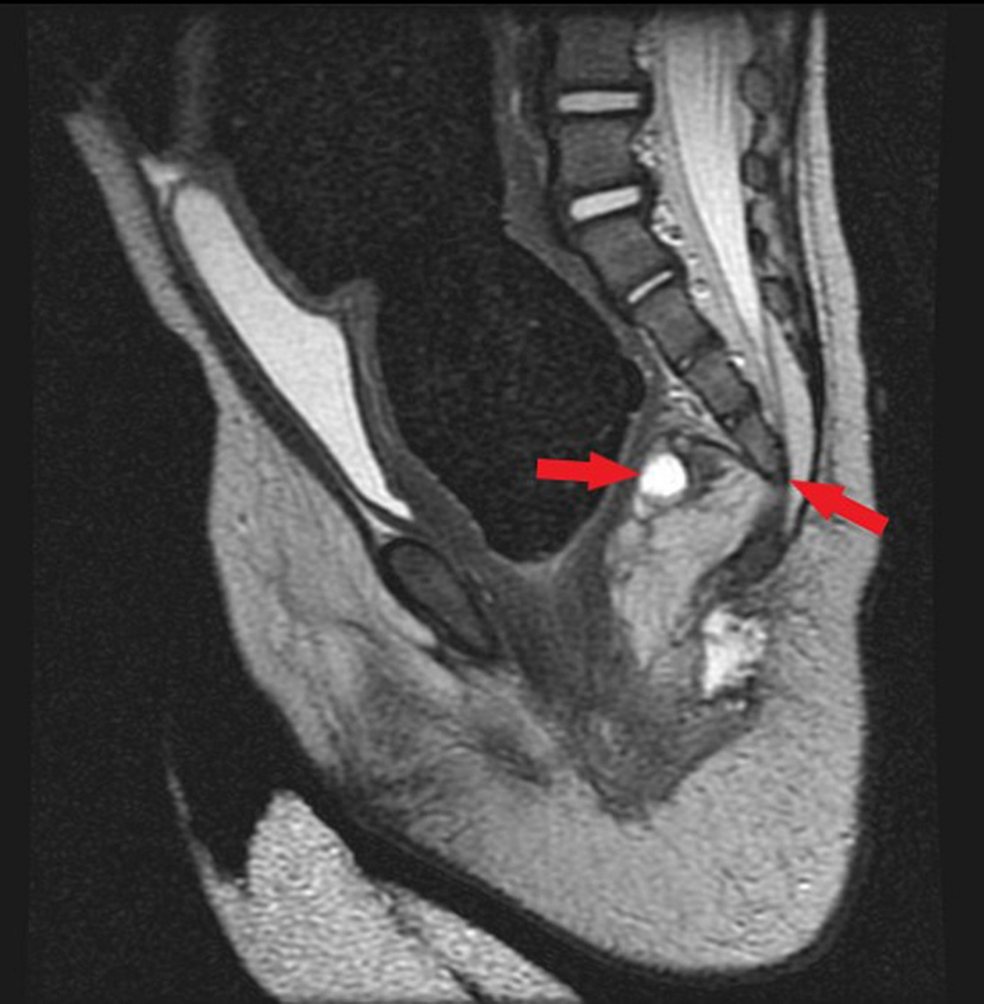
MRI showed there is the redemonstration of a defect at the anterior aspect of the sacrum opposite the S4/S5 level with herniation of a sizable lipoma into the presacral space measuring 2.8 x 2.2 x 3 cm (blue arrow) The distended colon is seen compressing and displacing the urinary bladder anteriorly and superiorly (yellow arrow). A sacrococcygeal osseous defect is detected (red arrow).
